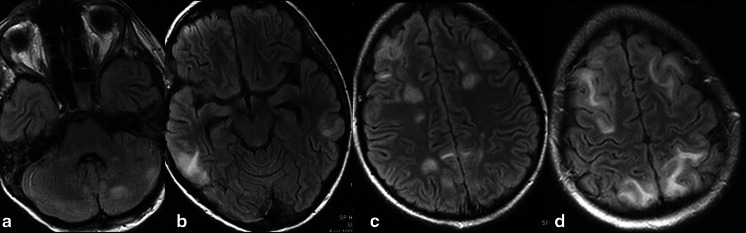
Case 1. a–d Axial T2-FLAIR images of the brain with hyperintensities in both cerebral hemispheres and the left cerebellar hemisphere
